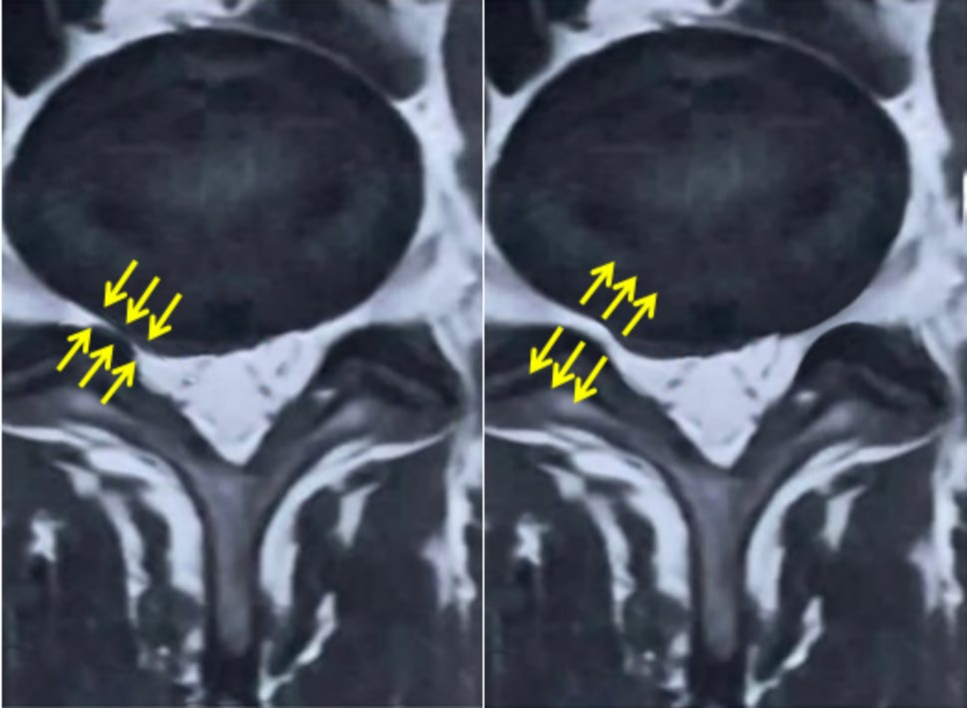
Lumbar MRI images, showing the LIVF at L5/S1. The right image depicts the modified reversed contralateral axial rotation (MRCAR) position, whereas the left depicts the supine position
